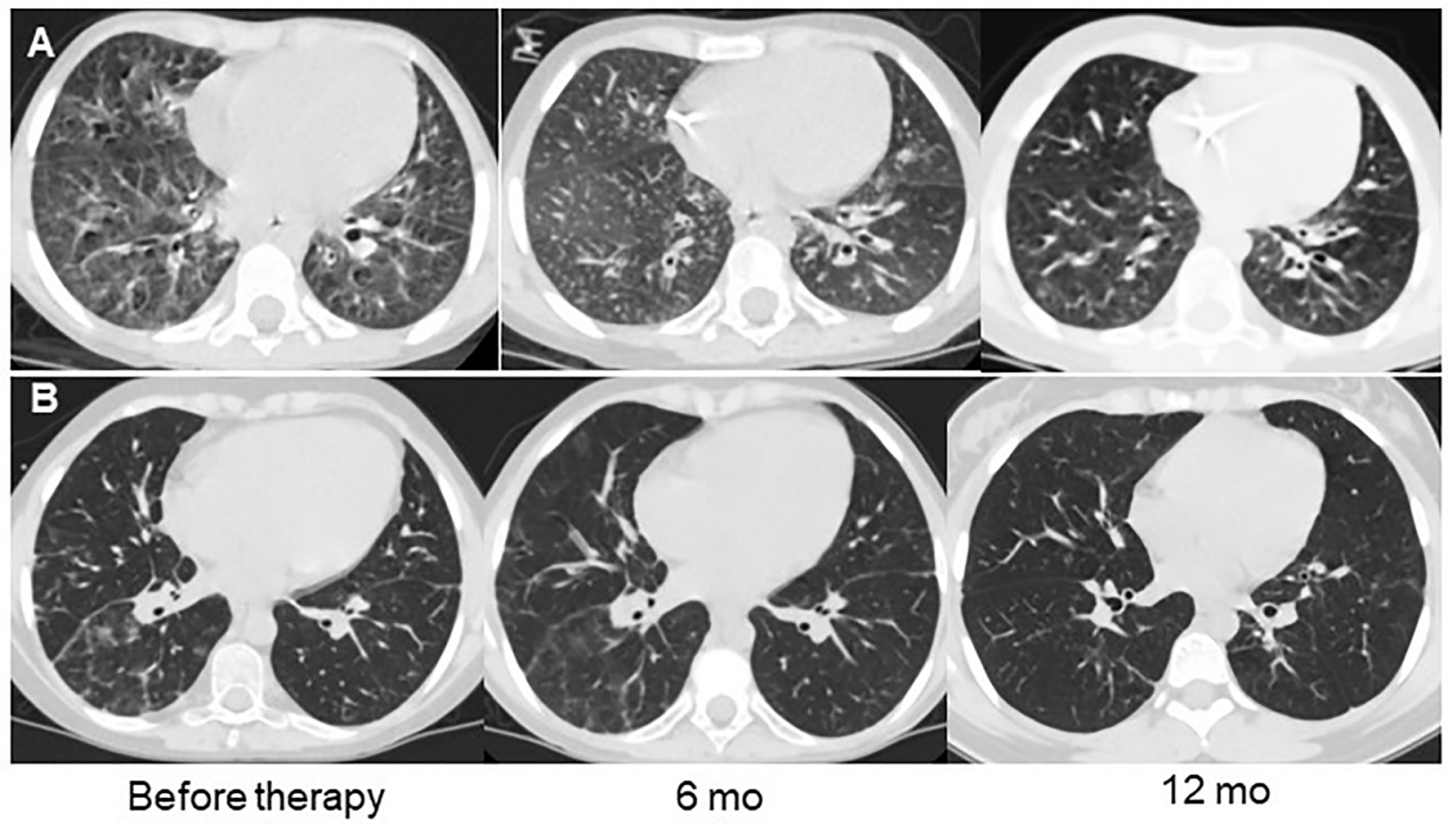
Examples of lung CT dynamics in the course of abatacept therapy. (A) – P28 with CTLA4 deficiency, (B) – P27 with ataxia-telangiectasia.
